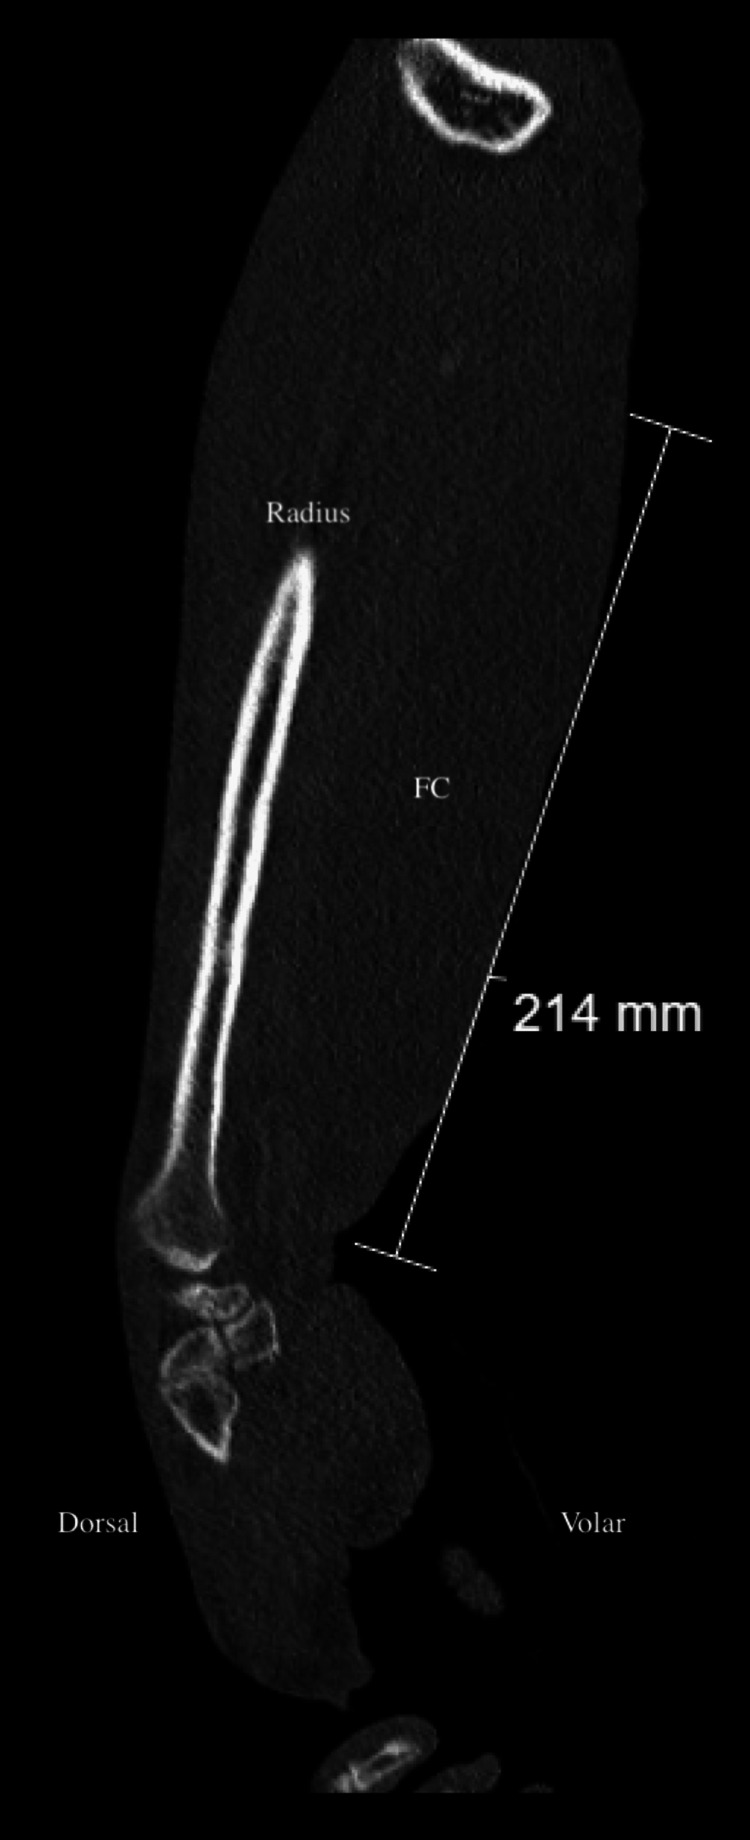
Imaging of the right forearm to evaluate the suspected Morel-Lavallée lesion (MLL). Contrast-enhanced computed tomography scan showing extensive soft tissue edema, though inconclusive due to motion artifacts, which prevented clear visualization of a defined lesion. FC: fluid collection.
